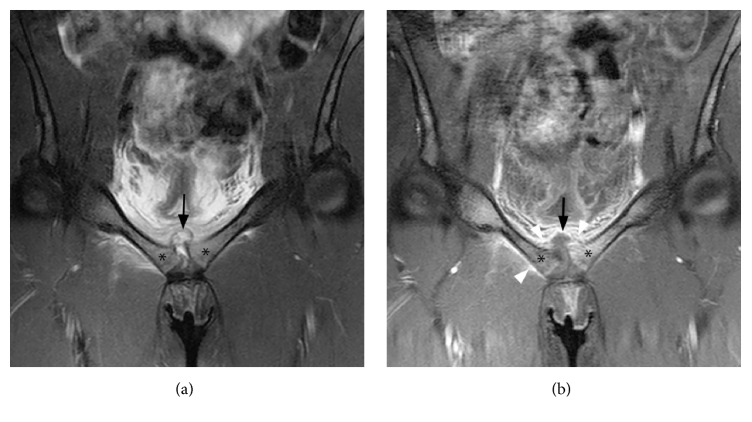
Corresponding coronal MR images of the pubic symphysis. (a) T2-weighted MR image with fat suppression showing bone marrow oedema in the pubic bones adjacent to the symphysis consistent with osteomyelitis (asterisk). A hyperintense fluid collection extending from the symphysis can be seen (black arrow). (b) T1-weighted MR image with fat suppression after intravenous Gadolinium administration showing marked contrast enhancement surrounding the hypointense fluid collection (black arrow) representing an abscess. There is also contrast enhancement representing inflammation in the surrounding soft tissues (white arrowheads) and within the pubic bones (asterisk).
